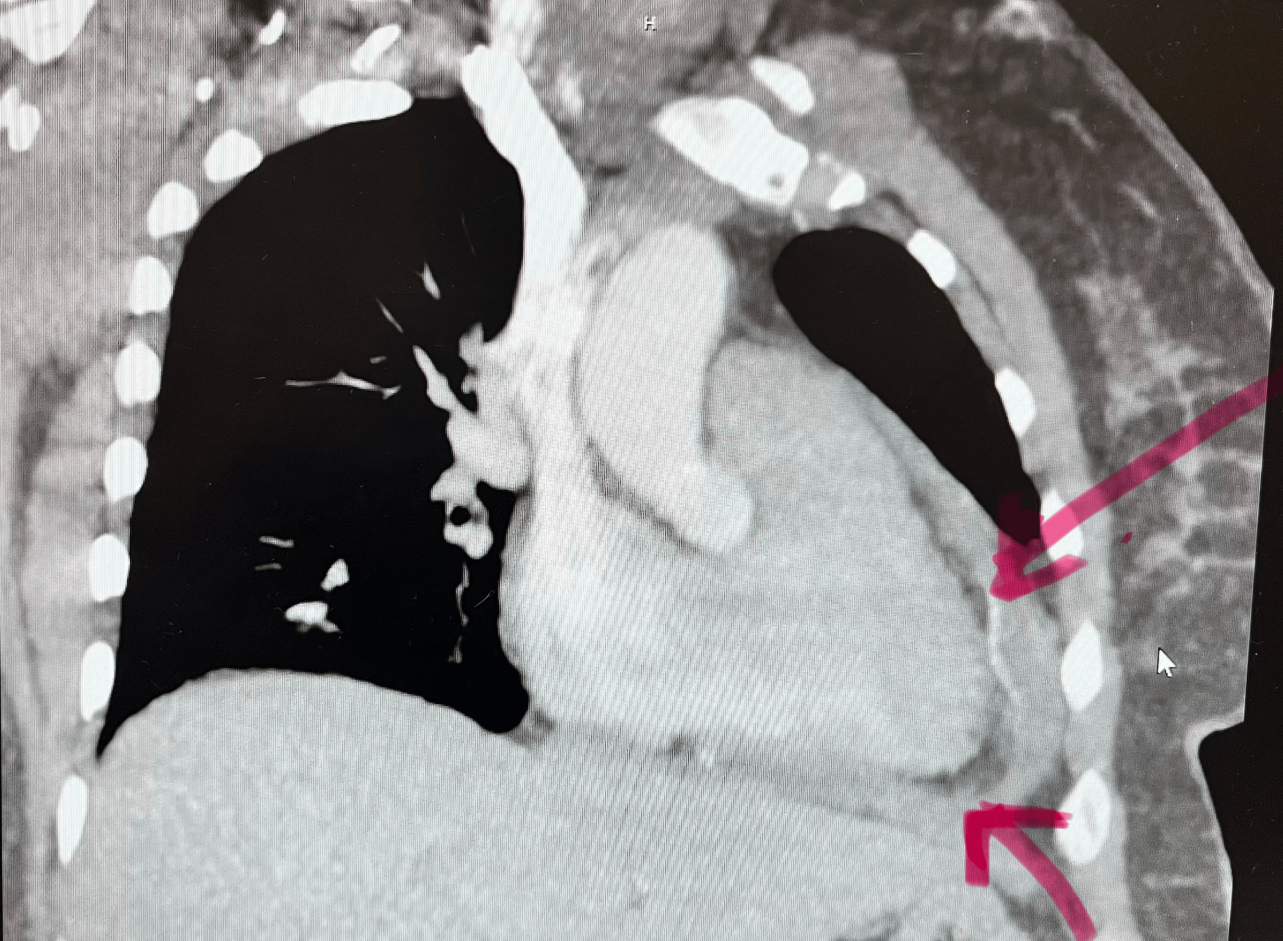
CT thorax showing pleural effusions with associated pericardial thickening CT: computed tomography
